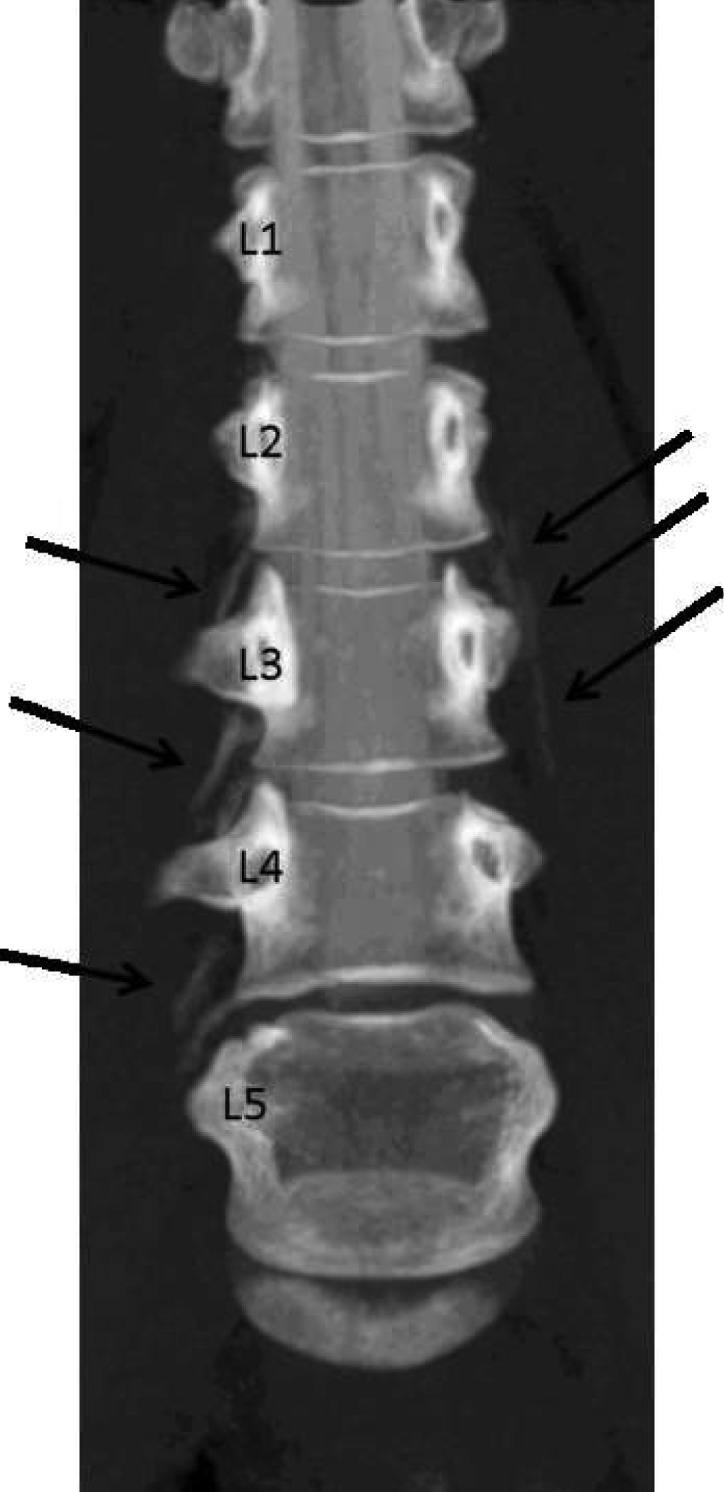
After injection of the contrast agent, CT scan was performed in prone position on a Philips Brilliance 40 CT-Scanner (Hamburg, Germany) in spiral mode, collimation 40 × 0.625 mm, 120 kV, 110 mAs. Multiplanar reconstructions were calculated. Time 16:13. Maximum intensity projection in anterior-posterior view showing the lumbar spine (lumbar vertebral bodies 1-5, see numbers). The contrast agent is now visible on both sides outside the subarachnoid space distributing parallel to the spinal nerves.
